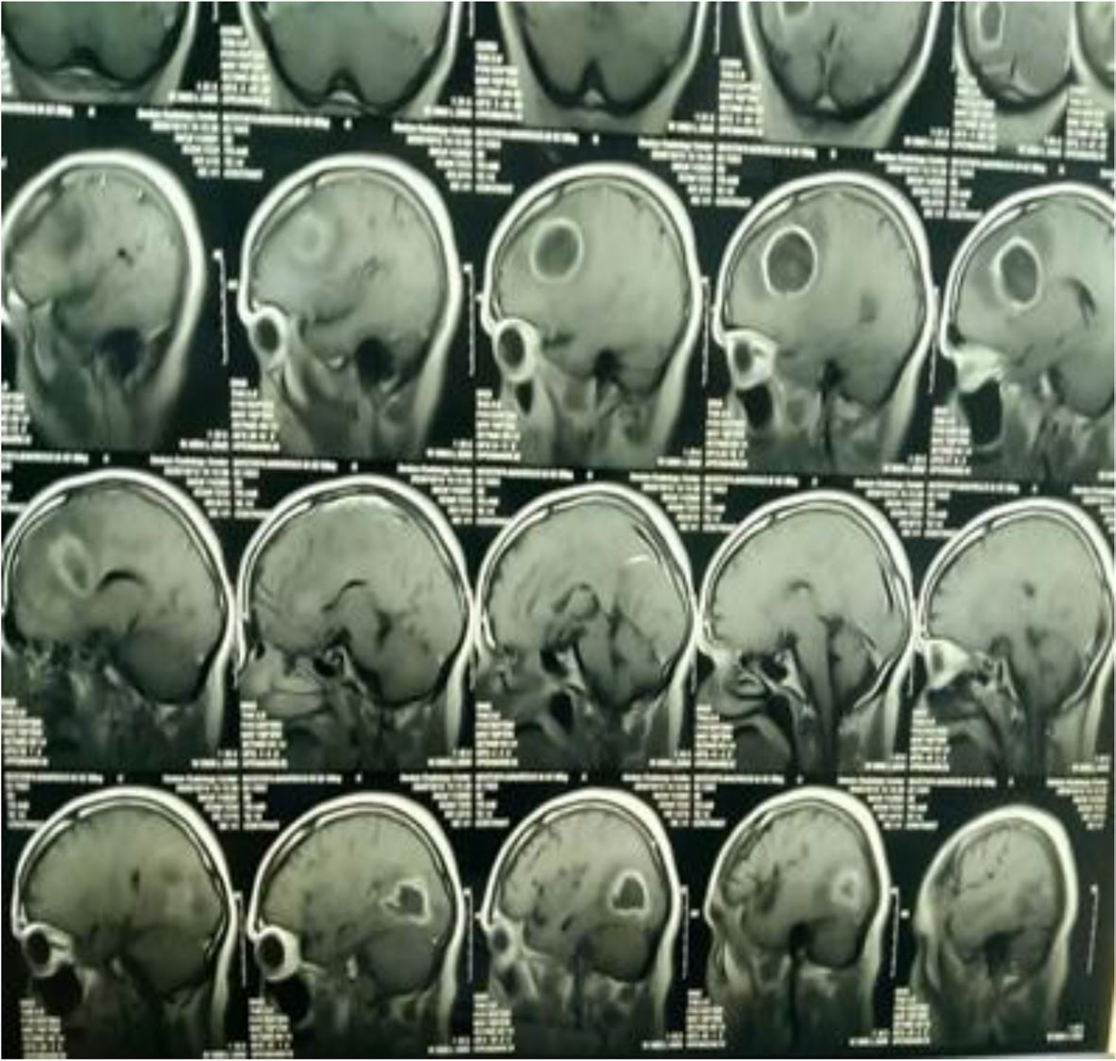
MRI with contrast sagittal and coronal sections show left frontal and right parietal—intra-axial hypodense brain lesions with a margin of enhancement
